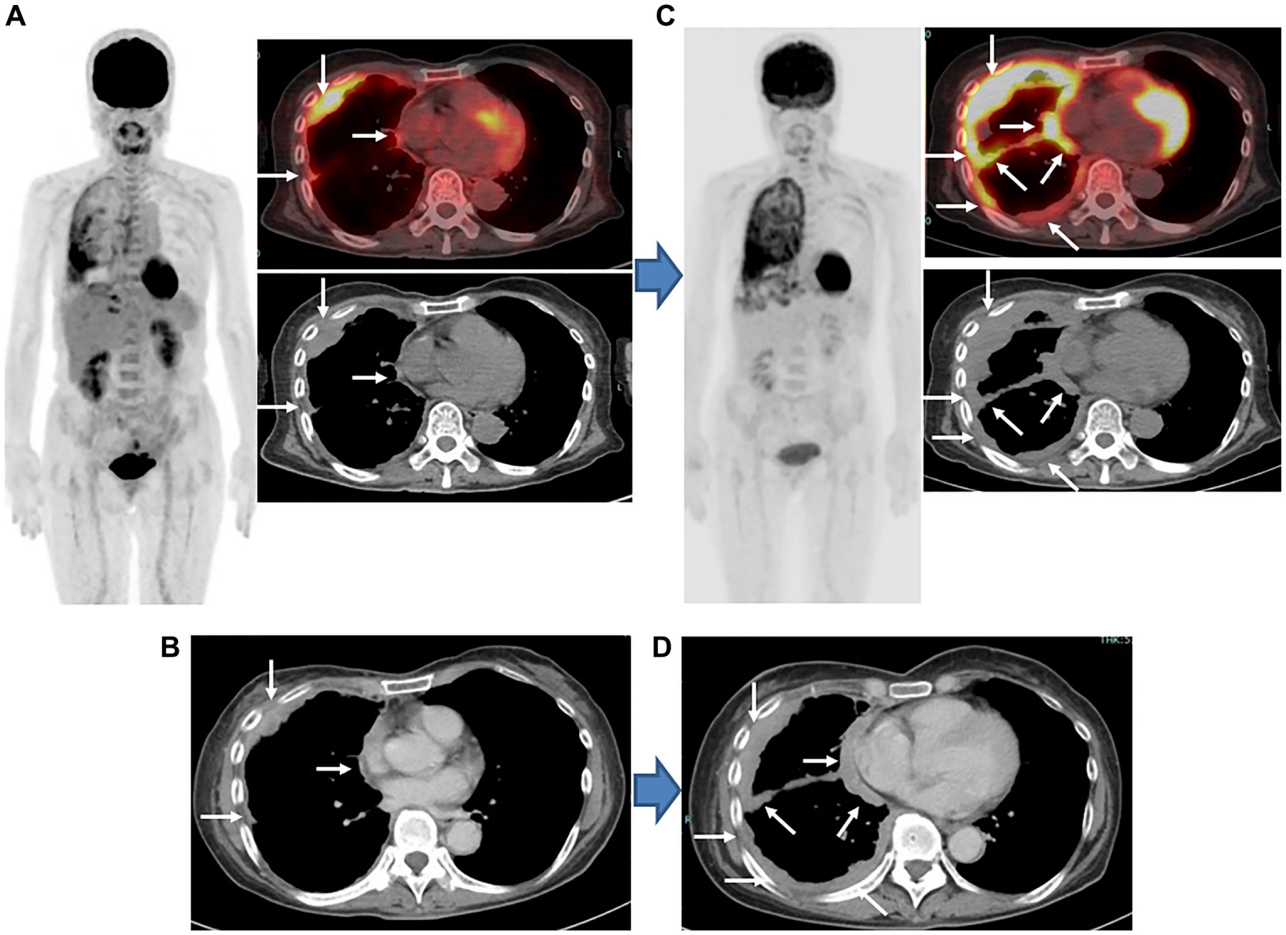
Epithelioid MPM, stage IIIB (cT4N0M0), woman, 64 years old. (A) Findings obtained with FDG-PET/CT prior to combined nivolumab and ipilimumab immunotherapy indicated several areas in right pleural lesions with strong FDG uptake (arrows). (B) Findings obtained with contrast-enhanced CT findings prior to combined nivolumab and ipilimumab immunotherapy indicated multiple areas of mass-forming thickness in right pleura (arrows). (C) Following three cycles of combined nivolumab and ipilimumab treatment, FDG-PET/CT findings showed remarkable progression of multiple pleural lesions and also revealed new pleural lesions (arrows). (D) Following three cycles of combined nivolumab and ipilimumab, contrast-enhanced CT findings also showed remarkable progression of pleural lesions and also revealed new lesions (arrows). imPERCIST findings showed PMD, while mRECIST indicated PD, due to remarkable progression and also new lesions. FDG-PET/CT results indicated an increase in SULpeak sum of 33.8% for the five highest level pleural lesions, while CT results showed an increase in the sum size of six pleural lesions perpendicular to the chest of 146.3%. Chemotherapy (cisplatin and pemetrexed) was started, though the patient died after 2.1 months.
